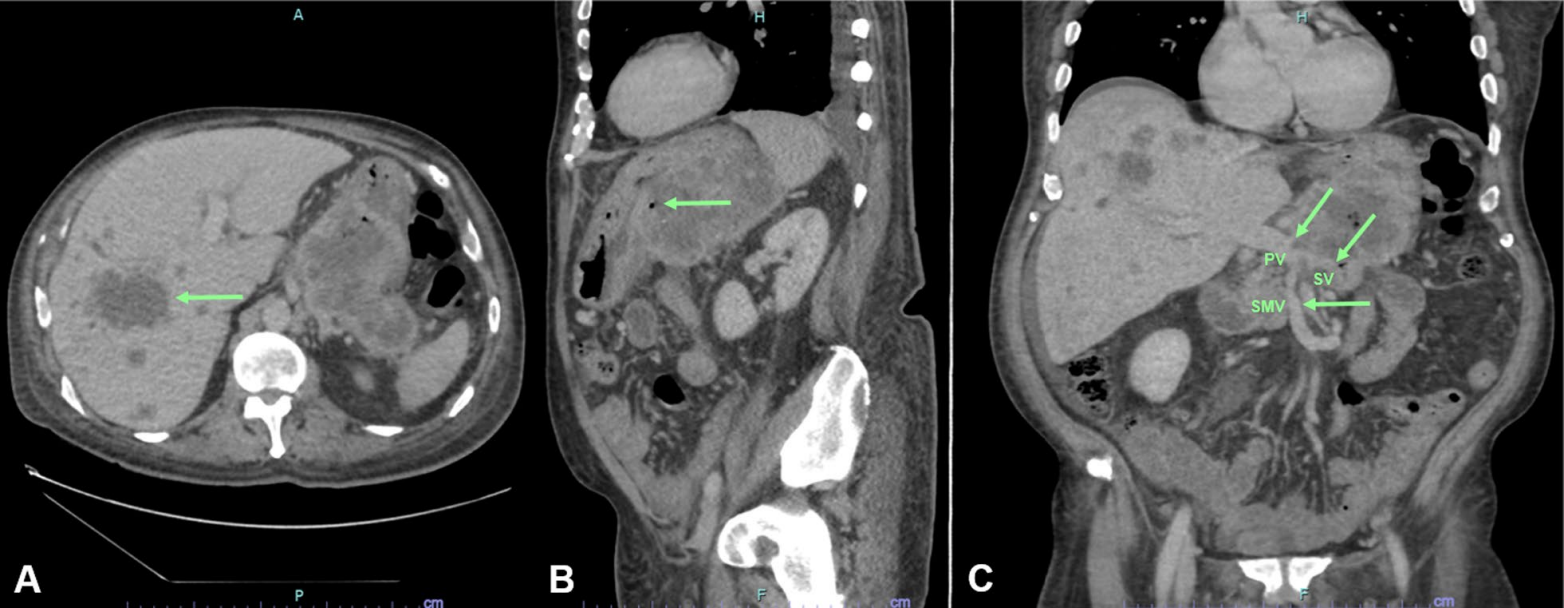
Abdominal pelvic CT scan showing a pancreatic body and tail SCC with multiple liver metastases. A Axial view demonstrating multiple hypoattenuating liver metastases with the largest lesion in segment 8 measuring 68 × 64 × 63 mm (arrow). B Sagittal view demonstrating the pancreatic mass compressing on the fundus and proximal gastric body with a small gaseous locule suggesting direct gastric invasion with possible fistulous formation (arrow), C coronal view showing the pancreatic SCC invading superior mesenteric vein (SMV), splenic vein (SV) and abutting the confluence of the portal vein (PV) by more than 180° (arrows)
